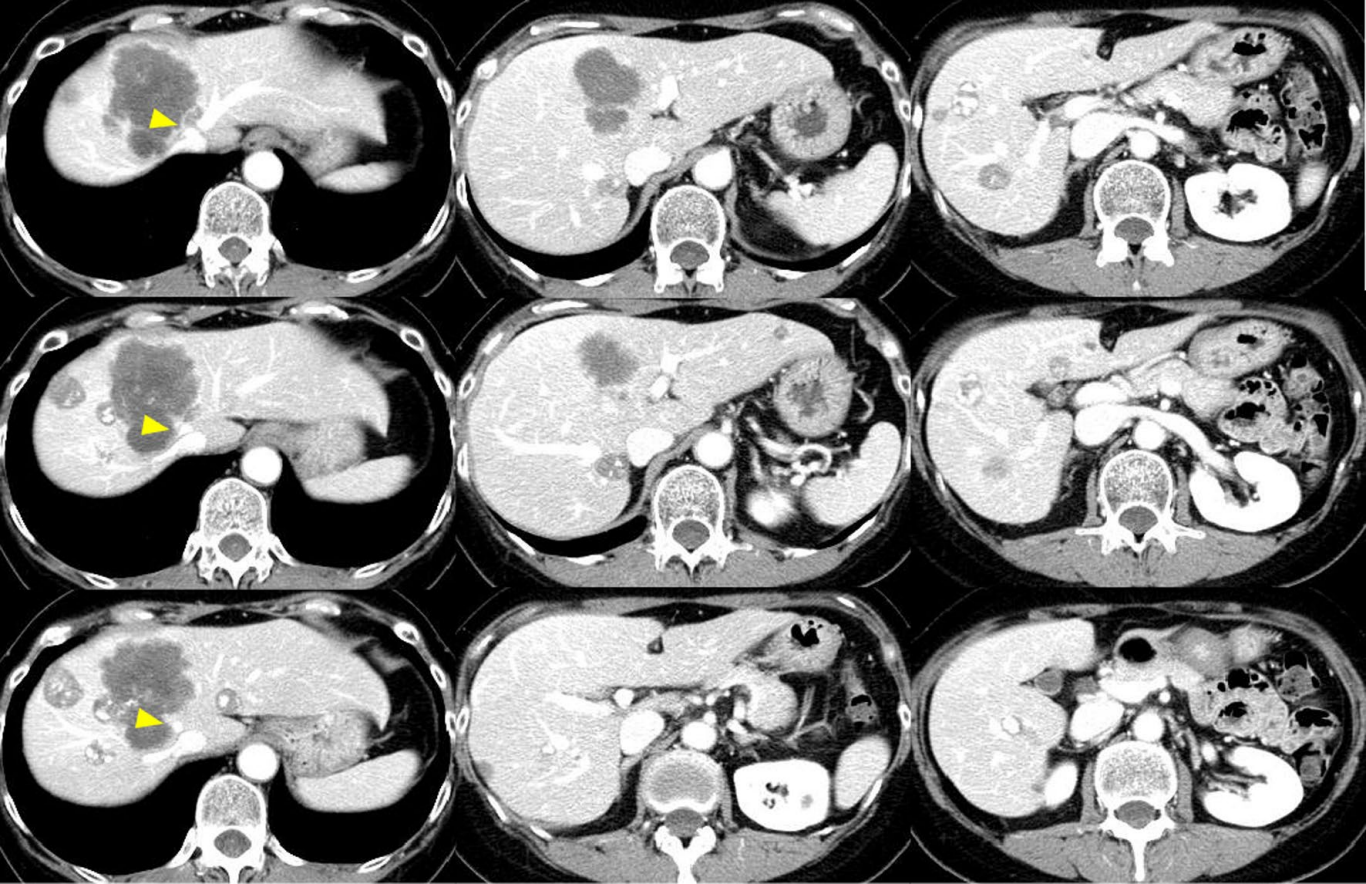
Computed tomographic findings after chemotherapy. While liver metastases are reduced overall, tumor involving segments 4 to 8 has invaded the MHV near the confluence of the MHV and the LHV (arrowhead)
